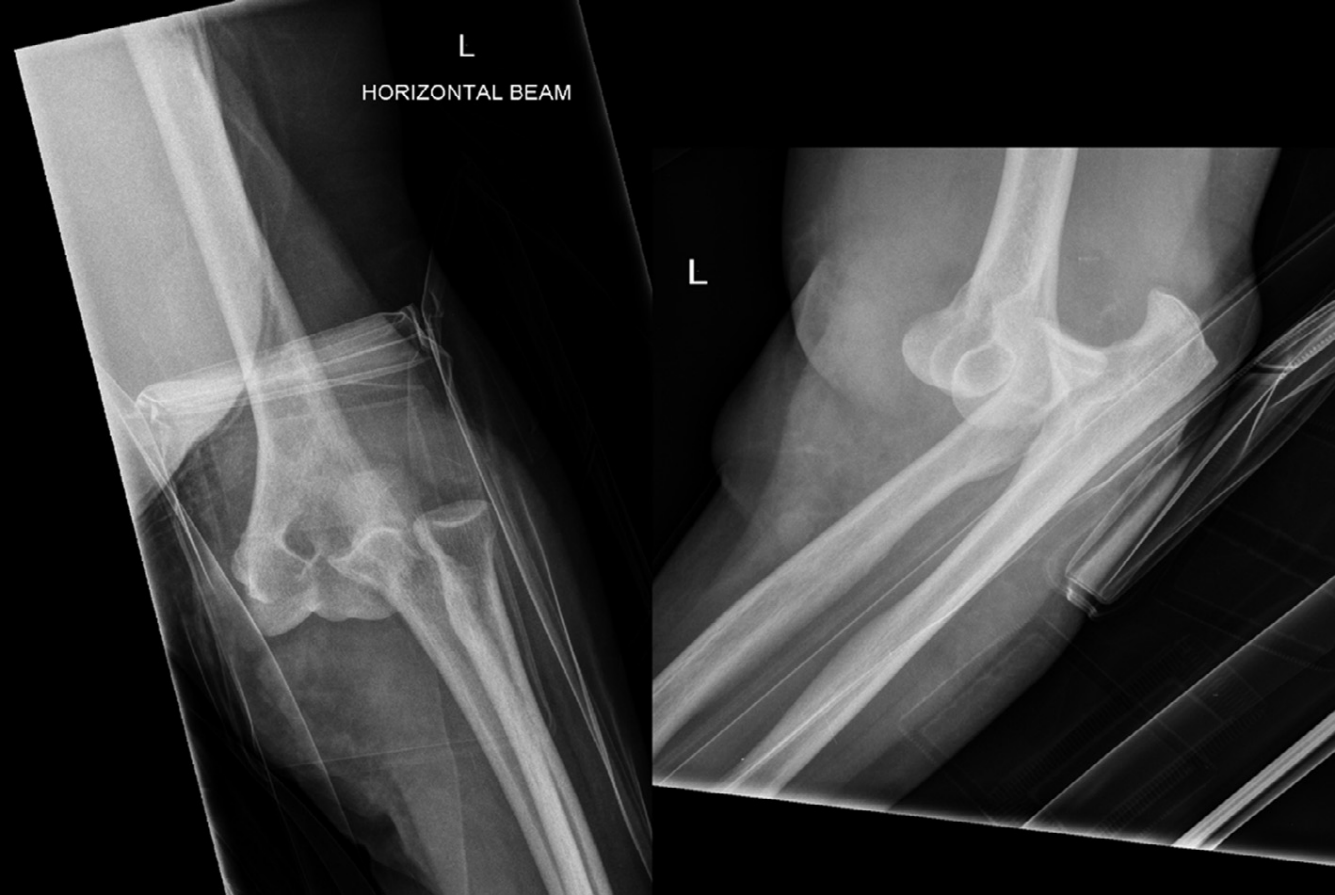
Elbow AP and lateral radiographs at presentation.
